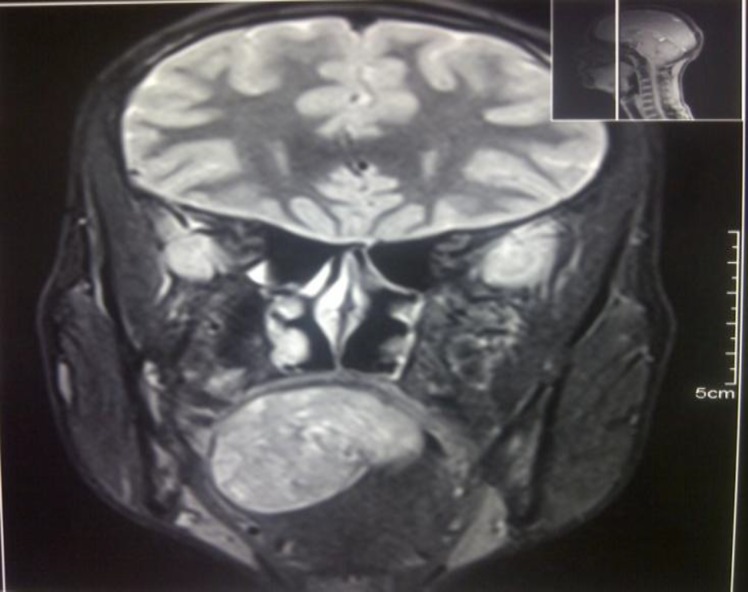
MRI study of the oral cavity showing the tongue lesion
